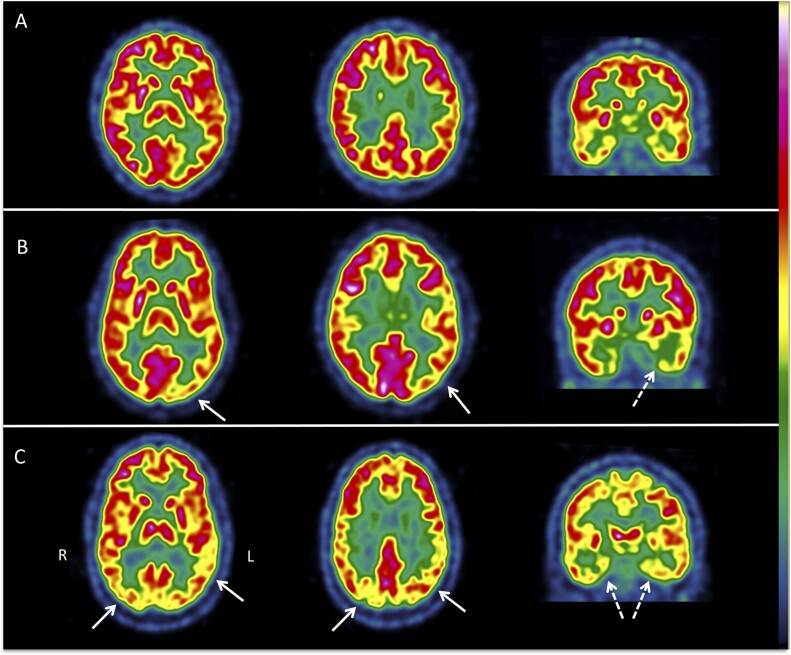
Example of brain FDG-PET/CT images in three patients with KLS. The images are displayed in axial and coronal views in radiological convention [right (R) is on the left (L)] with the French colour scale. Patient A, 18 y.o., had two episodes with hypersomnia, derealization, apathy and mood disturbances before the FDG-PET/CT. The cortical and subcortical metabolism was normal. Patient B, 17 y.o., had six episodes with hypersomnia, derealization, apathy, behavioural disturbances, hallucinations and psychiatric symptoms before the FDG-PET/CT scan. There was mild left temporo-occipital (white arrow) and hippocampal hypometabolisms (white dotted arrow). Patient C, 36 y.o., had 21 episodes with hypersomnia, derealization, apathy, behavioural disturbances and psychiatric symptoms. There was a bilateral associative parieto-temporo-occipital (white arrow) and hippocampal hypometabolism (white dotted arrow).
